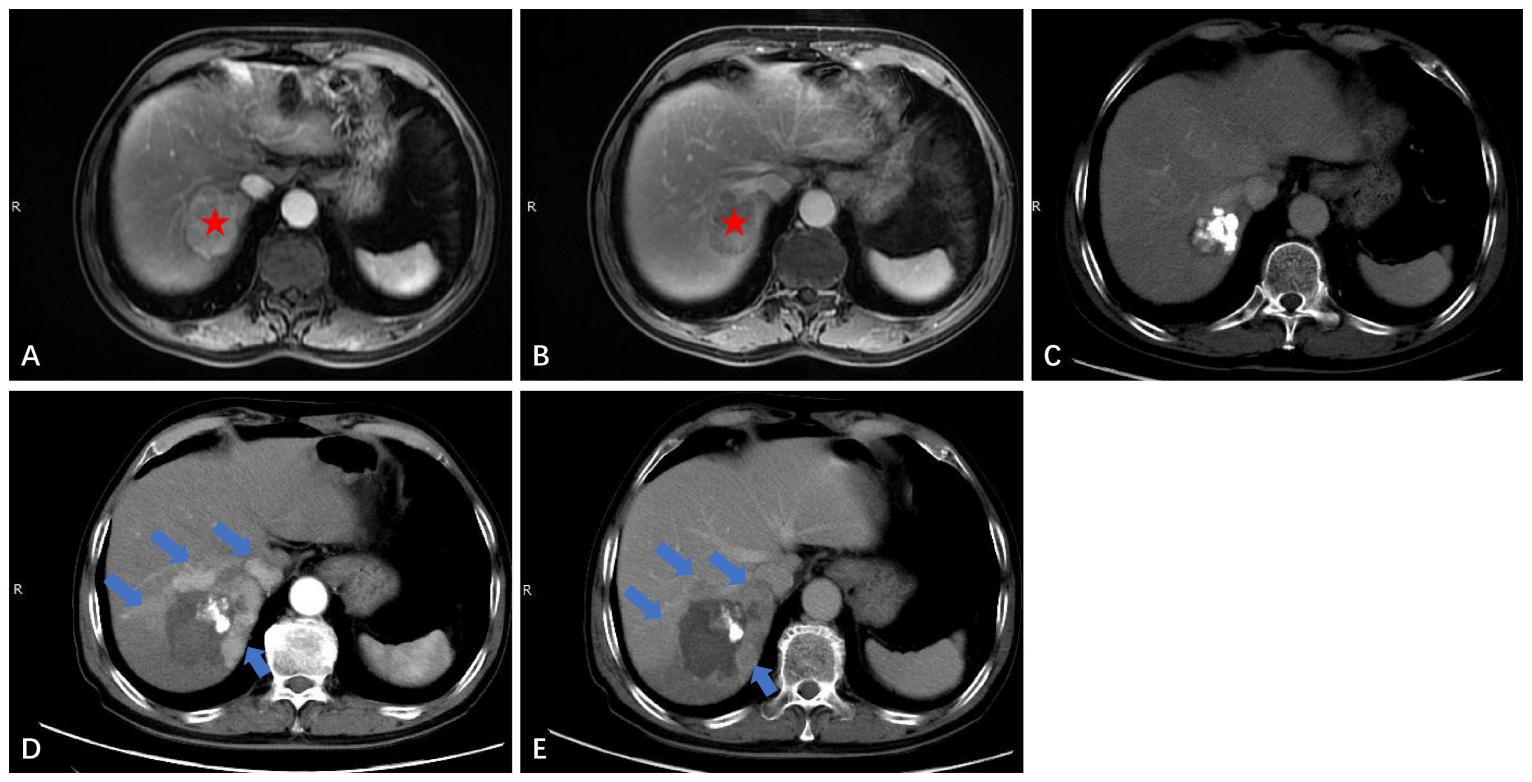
Local tumor progression (LTP) after TACE-RFA for HCC in a 72-year-old man. Finally, the patient received TACE as a second-line treatment. (A) Dynamic contrast-enhanced axial MRI scan shows a single 4.6cm×3.7cm×3.4cm HCC (red star). (B) MRI scan shows washout at the portal venous phase (red star). (C) CT scan obtained during the portal venous phase 1 month after TACE-RFA shows the complete ablation zone adjacent to the hepatic vein. (D, E) CT scan obtained during the hepatic arterial phase and portal venous phase 28 months after TACE-RFA shows the LTP, with multiple arterial enhancing nodules around the original ablation lesion and washout at the portal venous phase (blue arrows).
